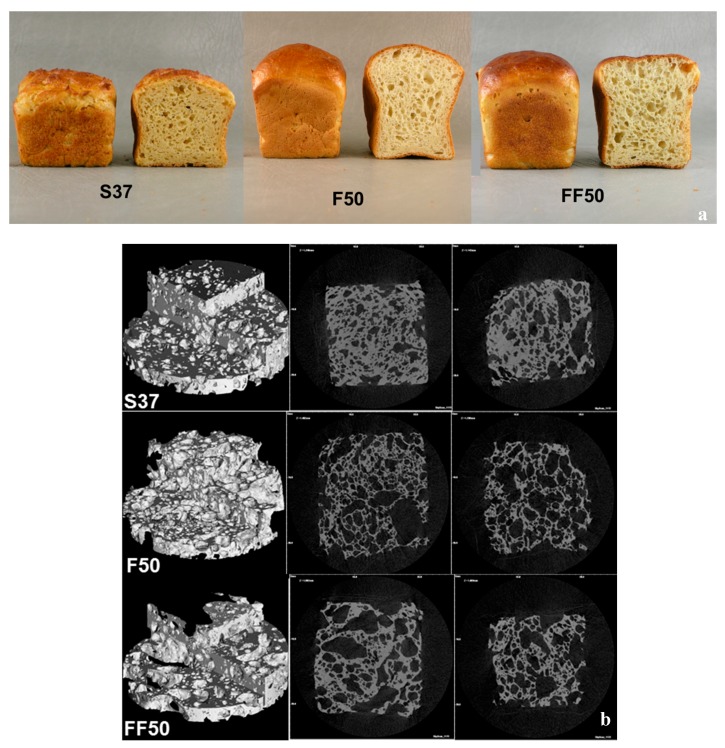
(a) Digital images of soy bread (S37), faba bread (F50), fermented faba bread (FF50). (b) Rendered three-dimensional (3D) images and selected 2D reconstructed X-ray microtomography (XMT) images of bread samples.
